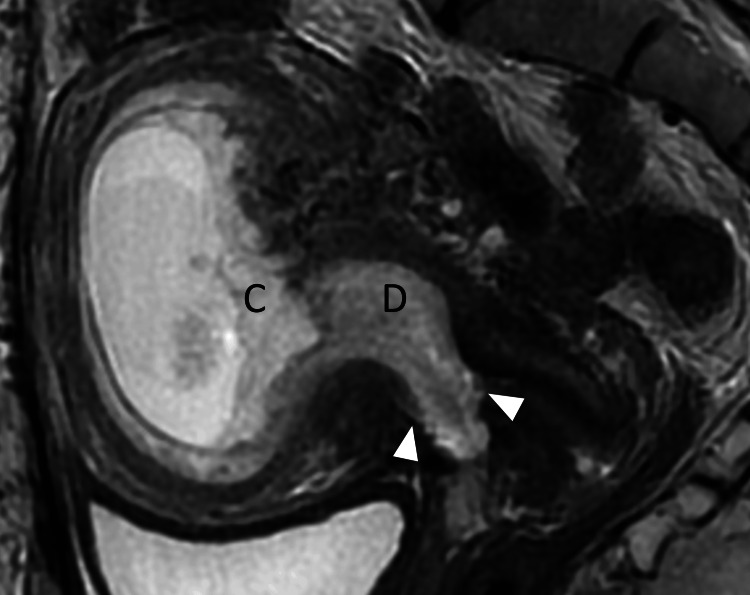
Sagittal T2WI MRI at 12 weeks gestation Absence of myometrium from the cervix to the anterior vaginal fornix (arrowhead). The myometrial defect site is observed to be infilled with decidual tissue (D), and chorionic villous tissue (C) is observed on the embryonic side of the defect, clearly separating the area from the decidual tissue. T2WI: T2 weighted image, MRI: magnetic resonance imaging
